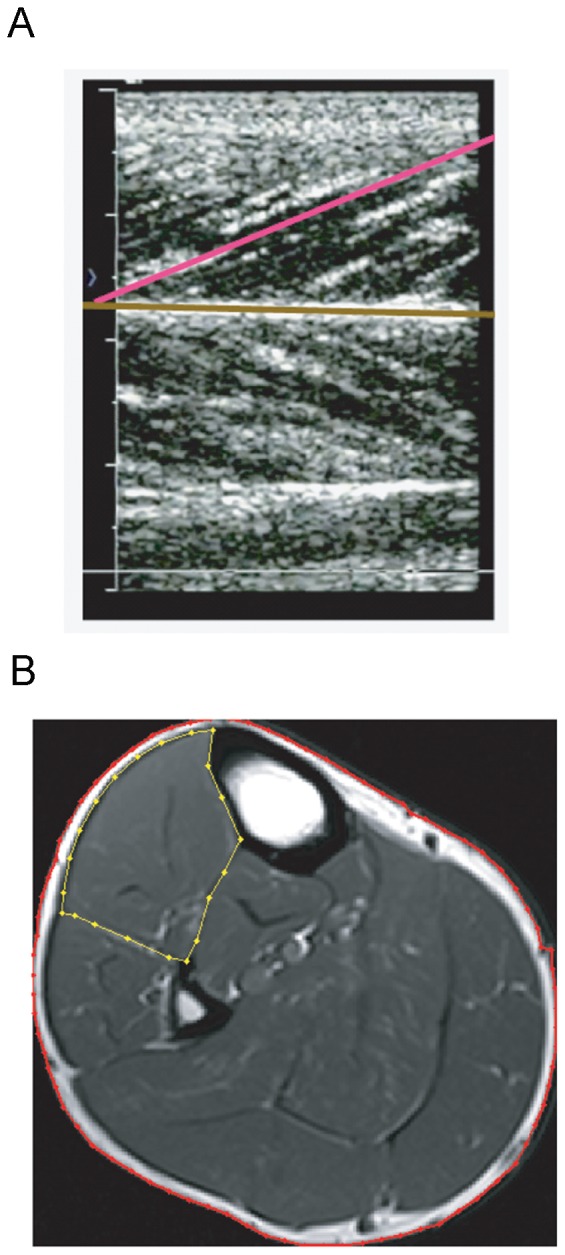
In vivo* Muscle Imaging. A: Ultrasound image of the anterior compartment of the lower limb. The horizontal line matches the orientation of the apponeurosis of the tibialis anterior muscle, and the angled line matches the pennation angle of visible fascicles under the ultrasound probe. B: Magnetic Resonance Image (MRI) in the Axial Plane. The dotted line represents the region of interest (ROI) for subsequent analysis of anterior compartment muscle size.
